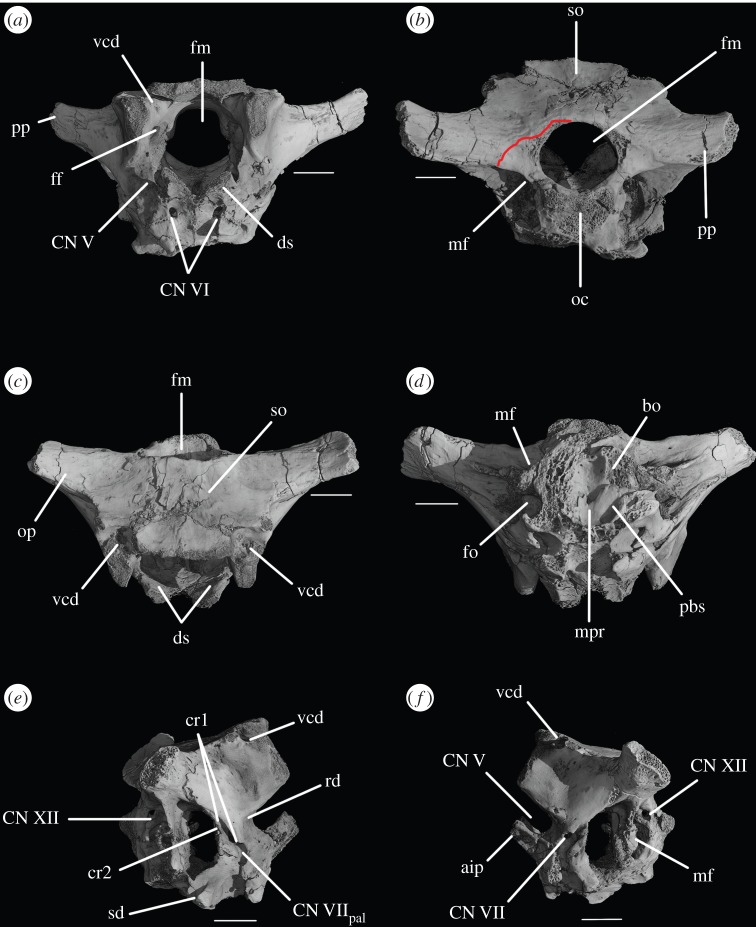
CT reconstruction of the braincase of SAM-PK-7696 in (*a*) anterior, (*b*) posterior, (*c*) dorsal, (*d*) ventral, (*e*) right lateral and (*f*) left lateral views. Red line in (*b*) indicates the suture line between exoccipital and opisthotic/supraoccipital based on CT scans (see figure 6). For abbreviations, see table 1.
